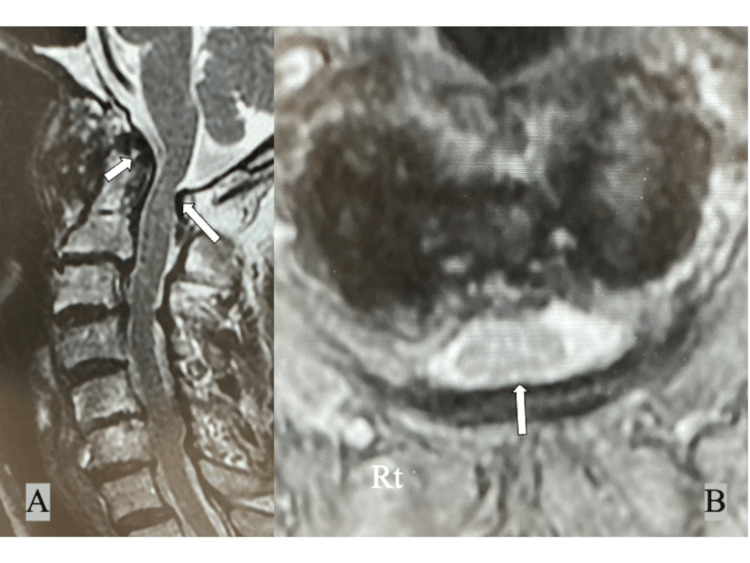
Preoperative MRI of the cervical spine showing (A) synovitis around the dens (white arrow) and (A, B) mild spinal cord compression between the dens and the posterior atlantal arch (white long arrows).
